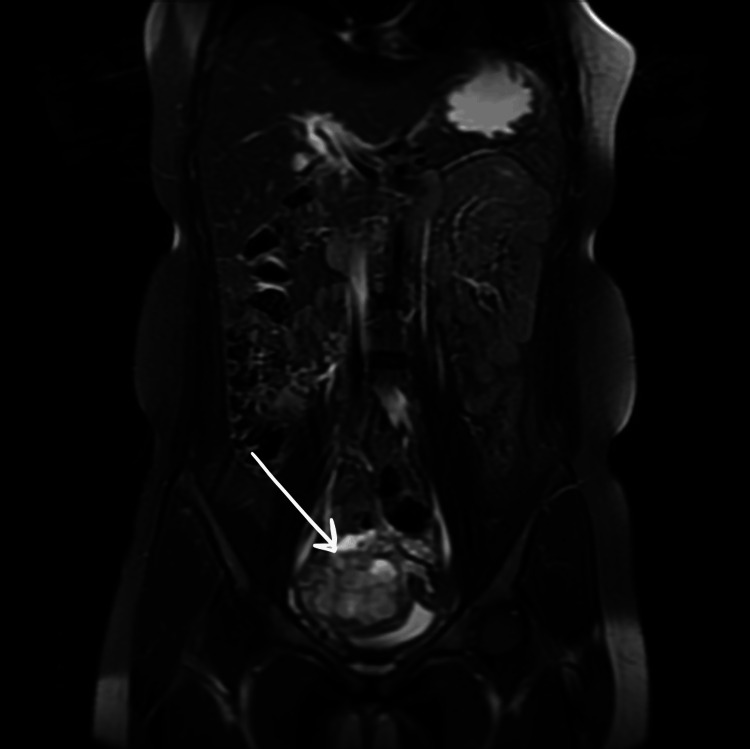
Coronal MRI (abdomen and pelvis) T2 fat-saturated sequence shows hyperintense lobulated soft tissue mass in the right hemipelvis arising from the urinary bladder.
